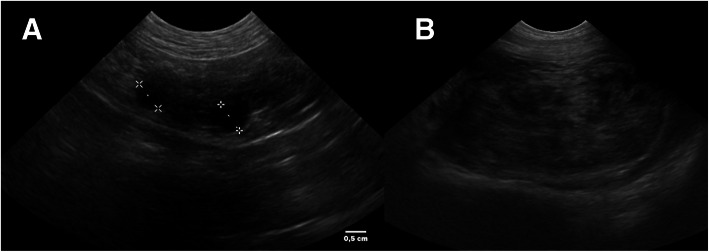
a Ultrasonography of the right ovary presenting preantral follicles of 0,7 cm. b Ultrasonography of the perineal area presenting the vaginal mass encapsulated in the cranial vagina
